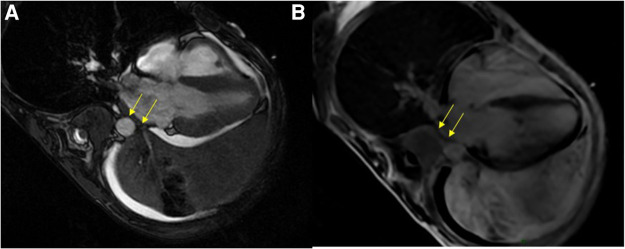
Cardiac MRI. SSFP four-chambers view showing the extensive tumoral process in the left pulmonary area coming into close contact with the left atrium, with paraneoplastic pleural and pericardial effusions (A); and likely tumoral invasion of the left atrial wall on the late-gadolinium-enhanced acquisition (B). MRI, magnetic resonance imaging; SSFP, steady-state free precession.
